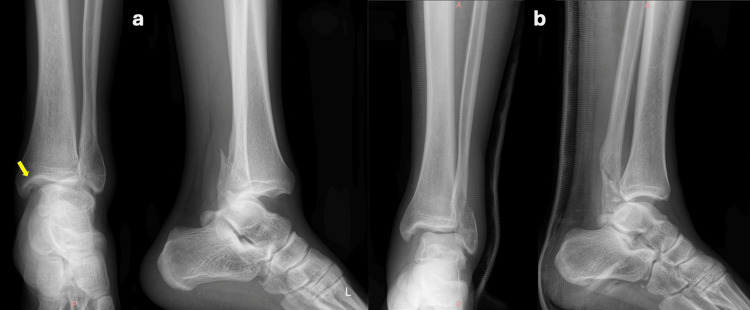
Pre-operative X-rays. (a) Initial anteroposterior and lateral x-rays revealing a left ankle joint dislocation with lateral malleolus fracture, unfortunately without forming a suspicion of a Bosworth injury (b) Closed reduction manipulations forced dislocated fibula to posterior angulation and failed to restore the anatomy causing increasing pain and discomfort to the patient in the next hours. The presence and the notability of the “axilla sign” (arrow) are controversial.
